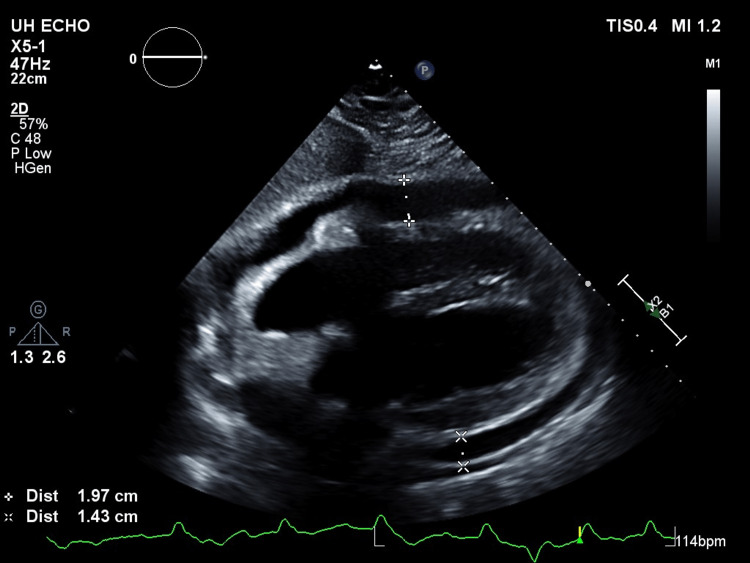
2-D echocardiographic parasternal long axis view of the heart with moderate to large circumferential pericardial effusion resulting in the beginnings of right ventricular collapse consistent with early cardiac tamponade physiology
